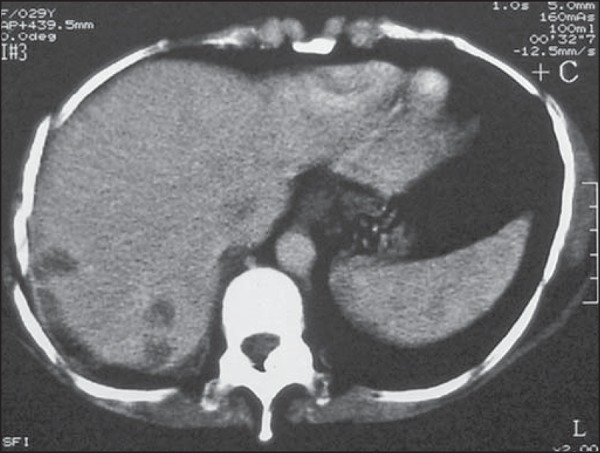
CT of the abdomen showing three hypointense, hypovascular lesions
